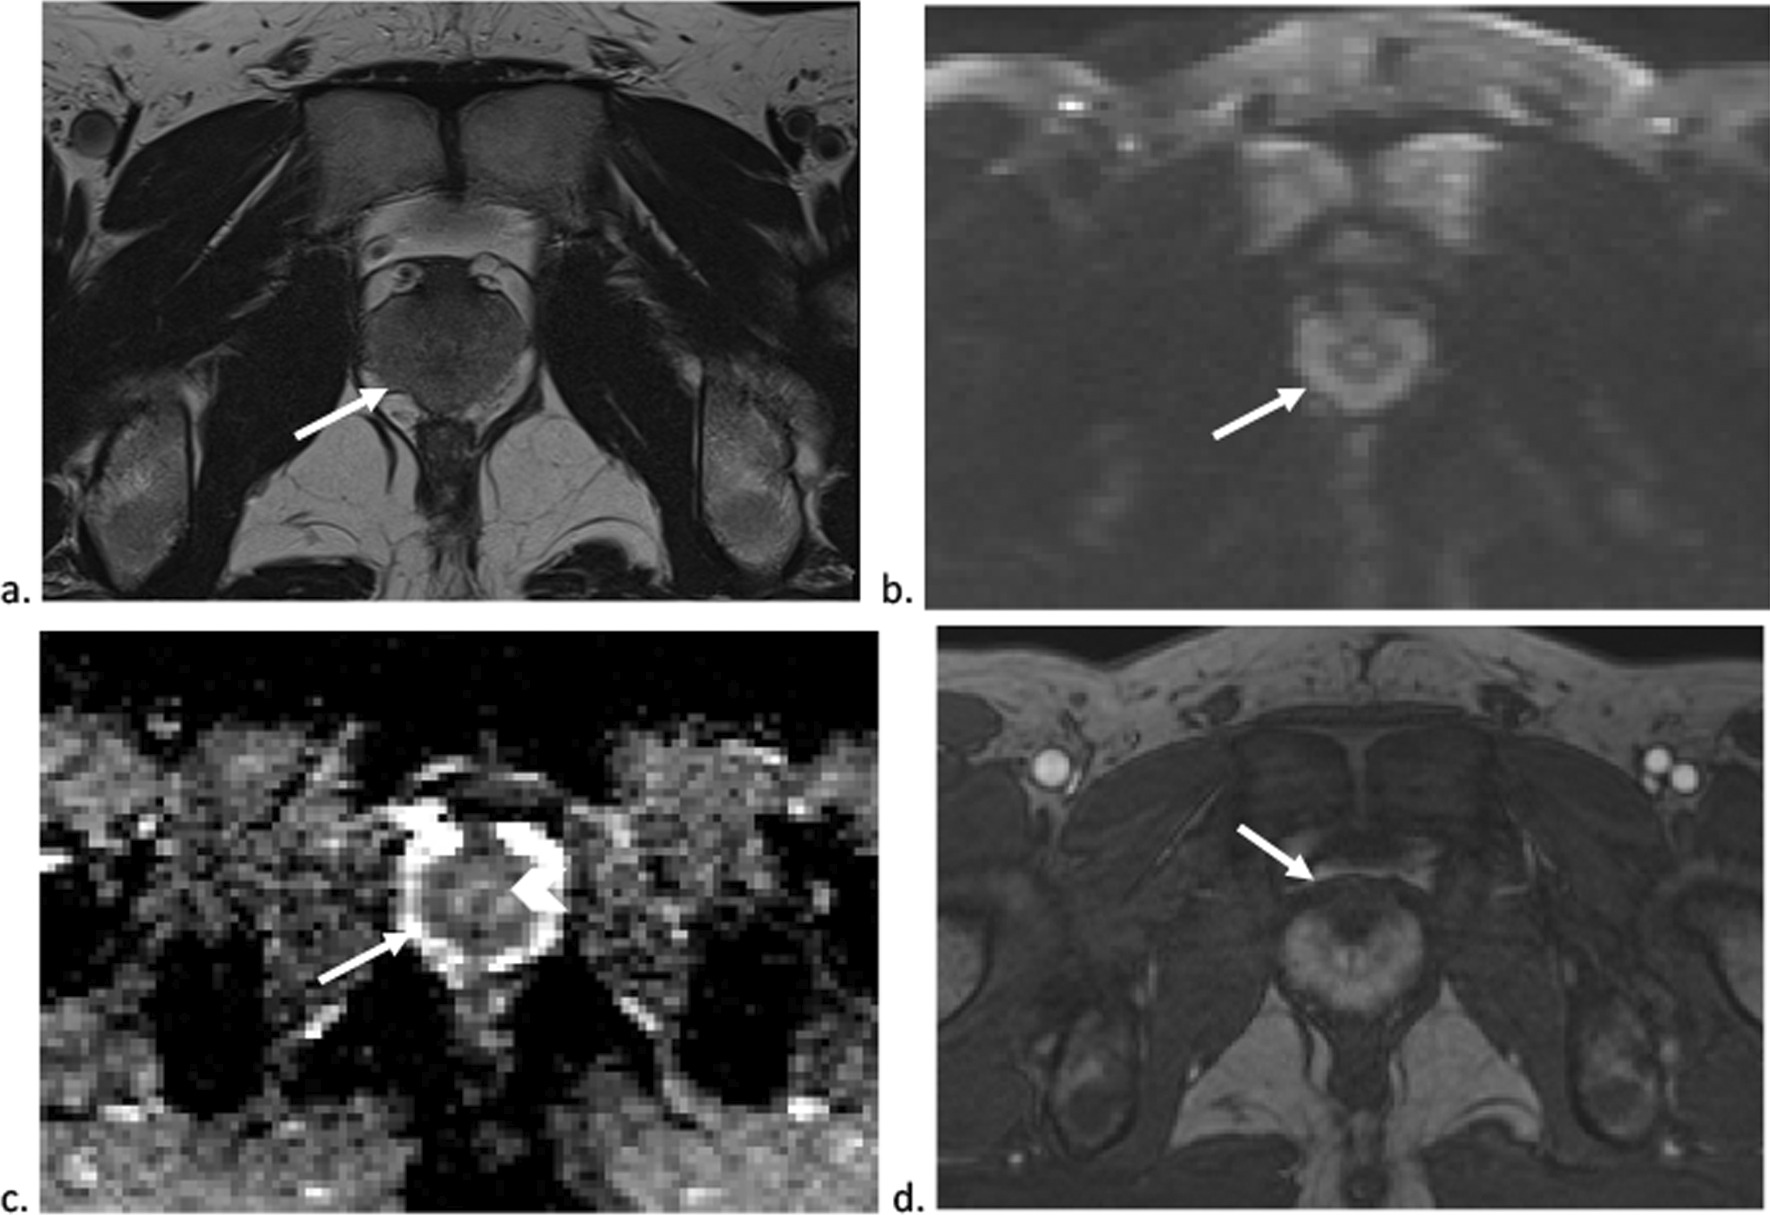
Follow-up MRI of the prostate gland four months later. T2 weighted (a), high b value 1500 DWI (b), ADC map (c) and dynamic contrast-enhanced (d) images show a decrease in size of the prostate gland with a volume of 24 cc (arrow). The peripheral zone demonstrates slightly more T2 hypointensity (a) (arrow) and slightly more restricted diffusion (c) (arrow). However, the transition zone has decreased in size and demonstrates less (c) restricted diffusion (arrowhead) and enhancement (arrow). The overall appearances are in keeping with resolving prostatitis
